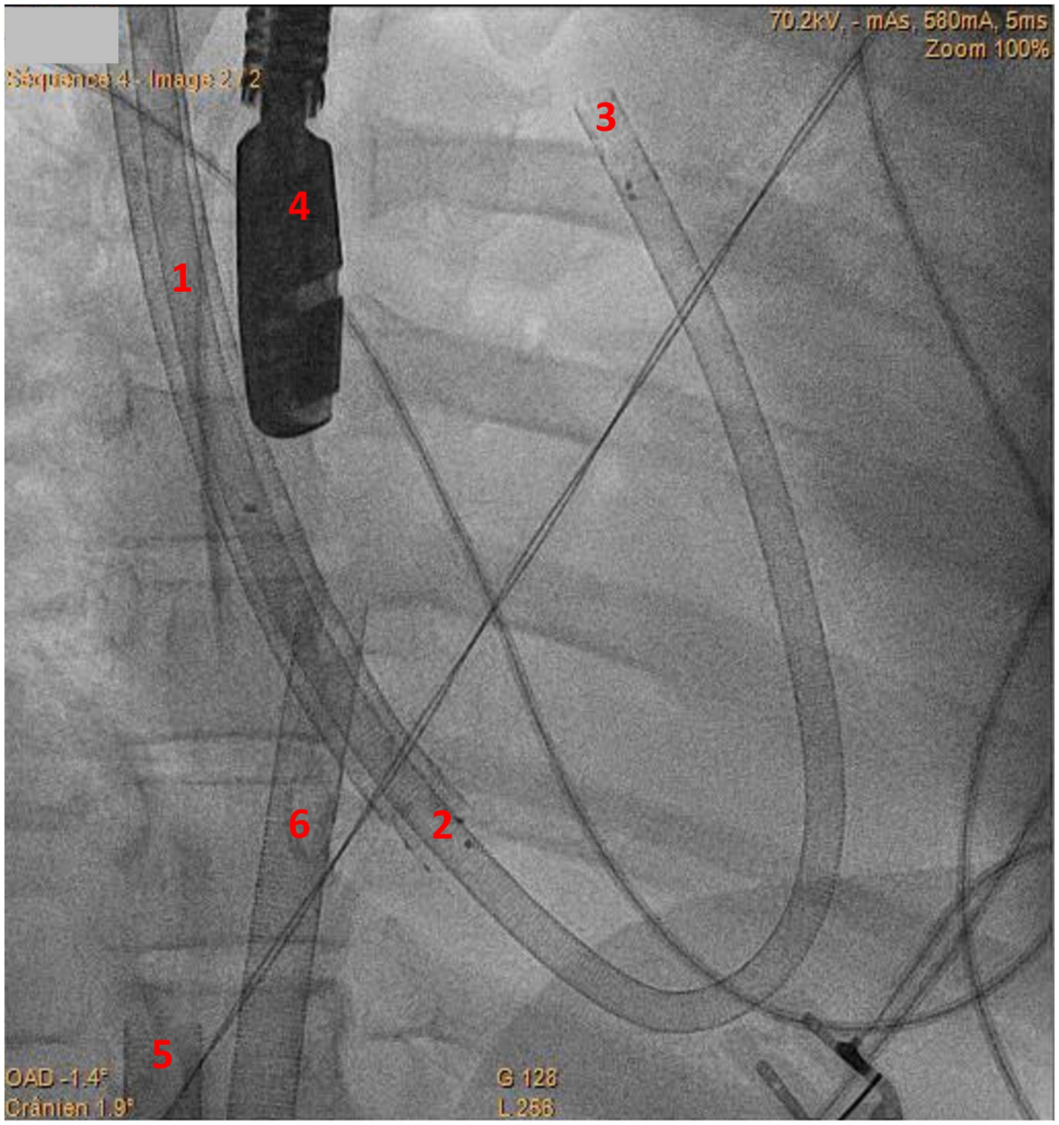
Fluoroscopy image of ProtekDuo cannula implantation. 1: ProtekDuo cannula in superior vena cava; 2: Inflow lumen of ProtekDuo cannula in place in right atrium; 3: tip of the ProtekDuo cannula (i.e., outflow lumen) in place in the main pulmonary artery; 4: tip of trans-esophageal echocardiography probe; 5: venous drainage cannula of femoro-femoral venovenous ECMO; 6: return cannula of femoro-femoral venovenous ECMO.
